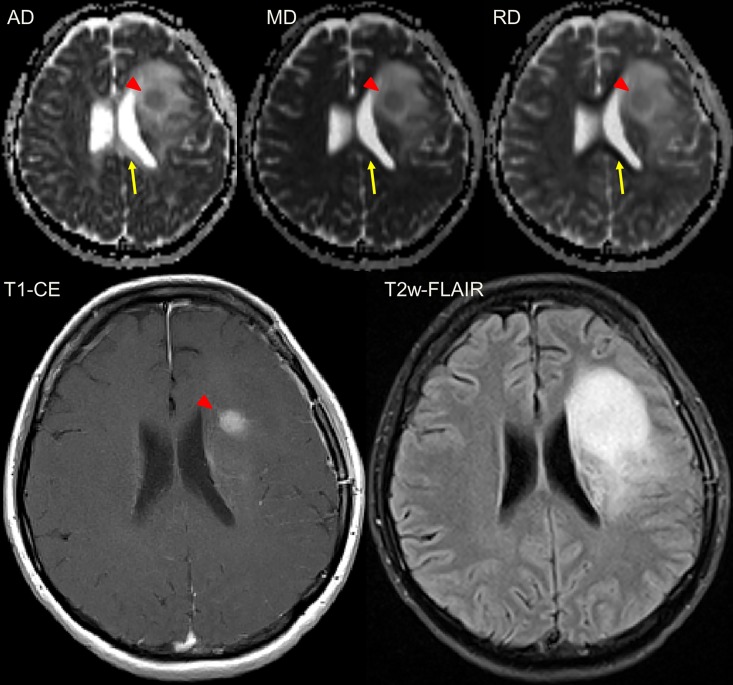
Female patient (43y) with a malignant transformation of a primary gemistocytic Astrocytoma WHO II. The transformation (red triangle) is visible in the T1w-CE images and at the same timepoint also in the diffusion tensor-derived parameter maps axial (AD), mean (MD) and radial diffusivity (RD). The area of changed brain tissue is most extensive in the T2w-FLAIR. Please note that only in the axial diffusivity map, white matter is depicted hyperintense (yellow arrow) which leads to difference in contrast. The T2 hyperintense region in the T2 FLAIR is matching with the hyperintense region in the DTI-parameter maps.
